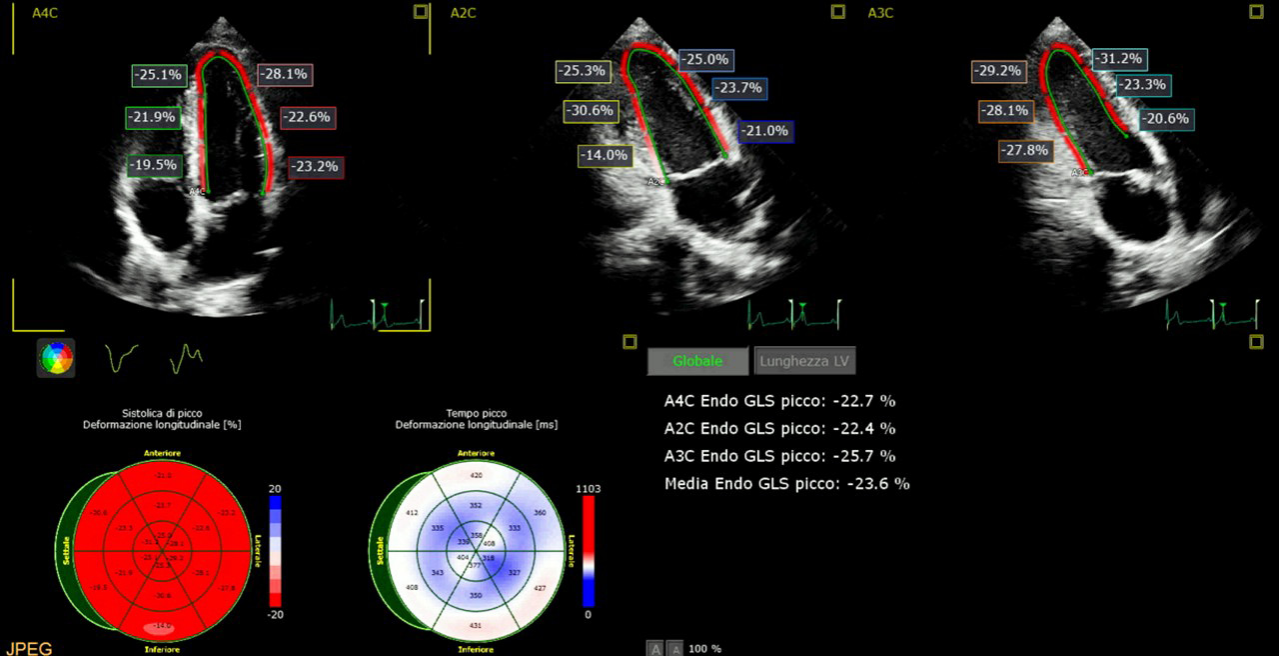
Echocardiographic analysis by speckle tracking: global longitudinal strain (GLS) control 6 months after diagnosis (−23%).
